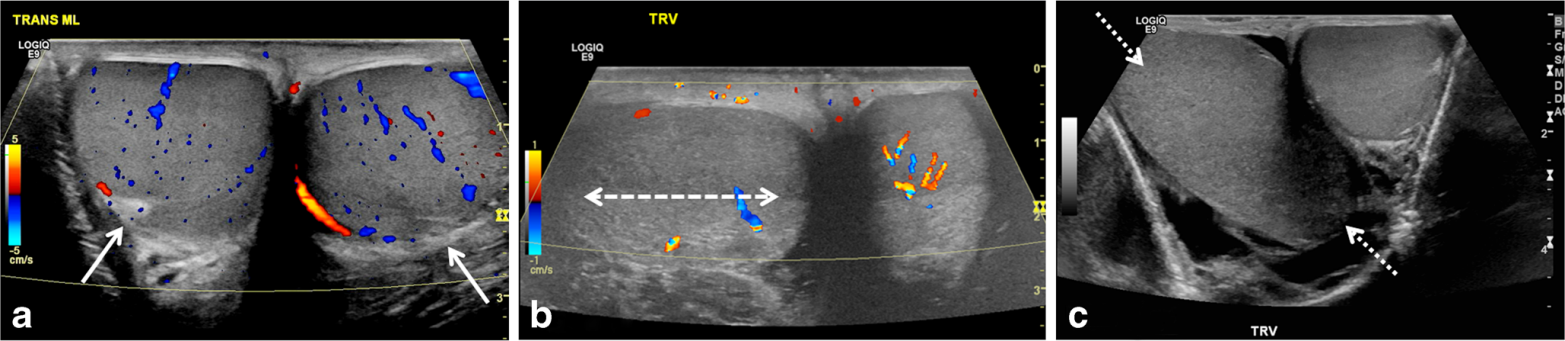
Testicular lie — normal and abnormal. a Color Doppler transverse US image of the testes in a 12-year-old boy with mild groin pain demonstrates normal vertical lie with the testes seen in round cross-sections and with the mediastinum testis (*arrows*) directed posterolaterally. b Color Doppler transverse US image of both testes in a 14-year-old boy who woke with acute right scrotal pain demonstrates abnormal horizontal lie of the right testis (*arrow*) with slightly decreased intratesticular flow compared to the normal left side. He had experienced similar episodes of pain in the past and was diagnosed with intermittent torsion. During orchiopexy 12 h later, a bell clapper deformity was noted bilaterally. c Gray-scale transverse US image of both testes in a 16-year-old boy with right testicular pain demonstrates abnormal oblique lie of the right testis (*arrows*), which is oriented diagonally compared to the normal left side. Intermittent torsion was diagnosed intraoperatively
